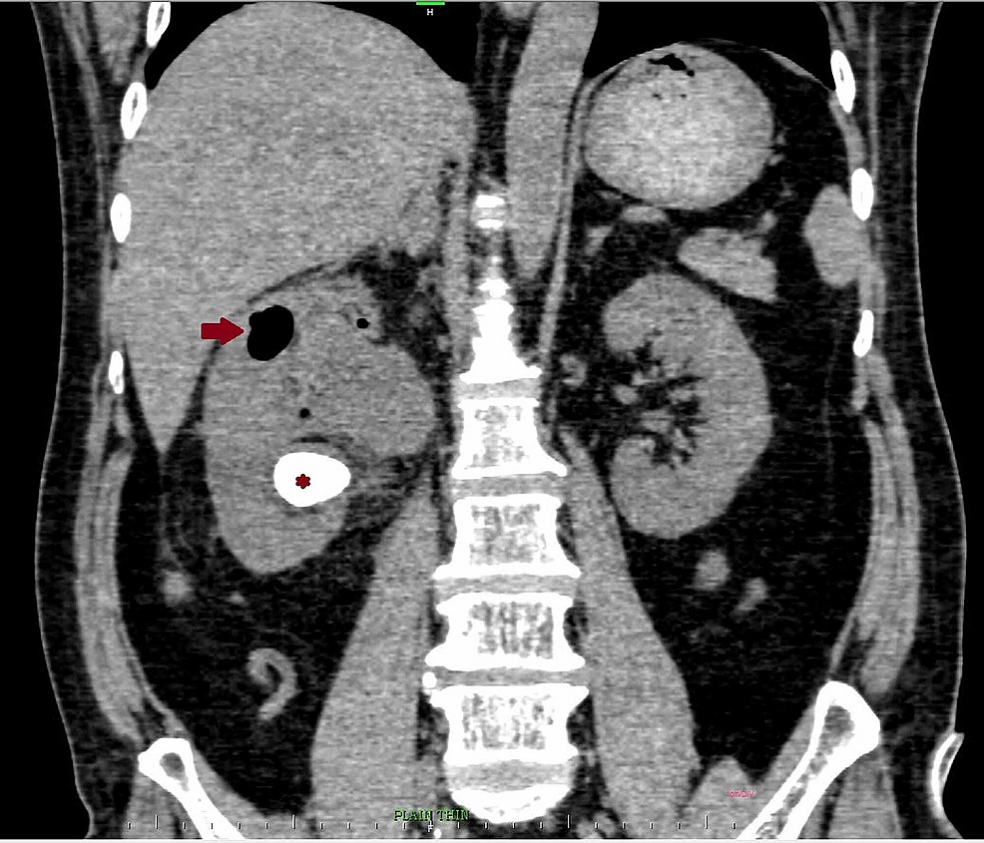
Sagittal reconstruction of CT abdomen revealed a large air pocket (red arrow) near the superior pole of the kidney. The air pocket was seen extending as a smaller pocket into the renal cortex, that was giving rise to emphysematous pyelonephritis. A large renal calculus was noted extending towards the lower pole (red asterisk).
